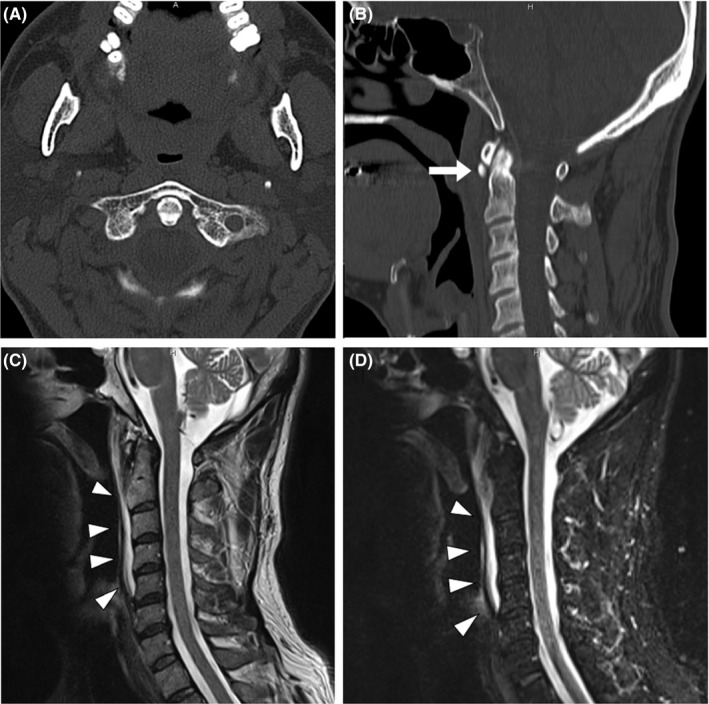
A, Cervical spine CT with horizontal slices in bone window revealed no finding of calcification around odontoid process; B, cervical spine CT with sagittal slices revealed calcification anterior to the odontoid process; C, T2‐weighted image in cervical MRI revealed prevertebral hyperintense area of edema at the C1‐C5 vertebral bodies; D, STIR image in cervical MRI
